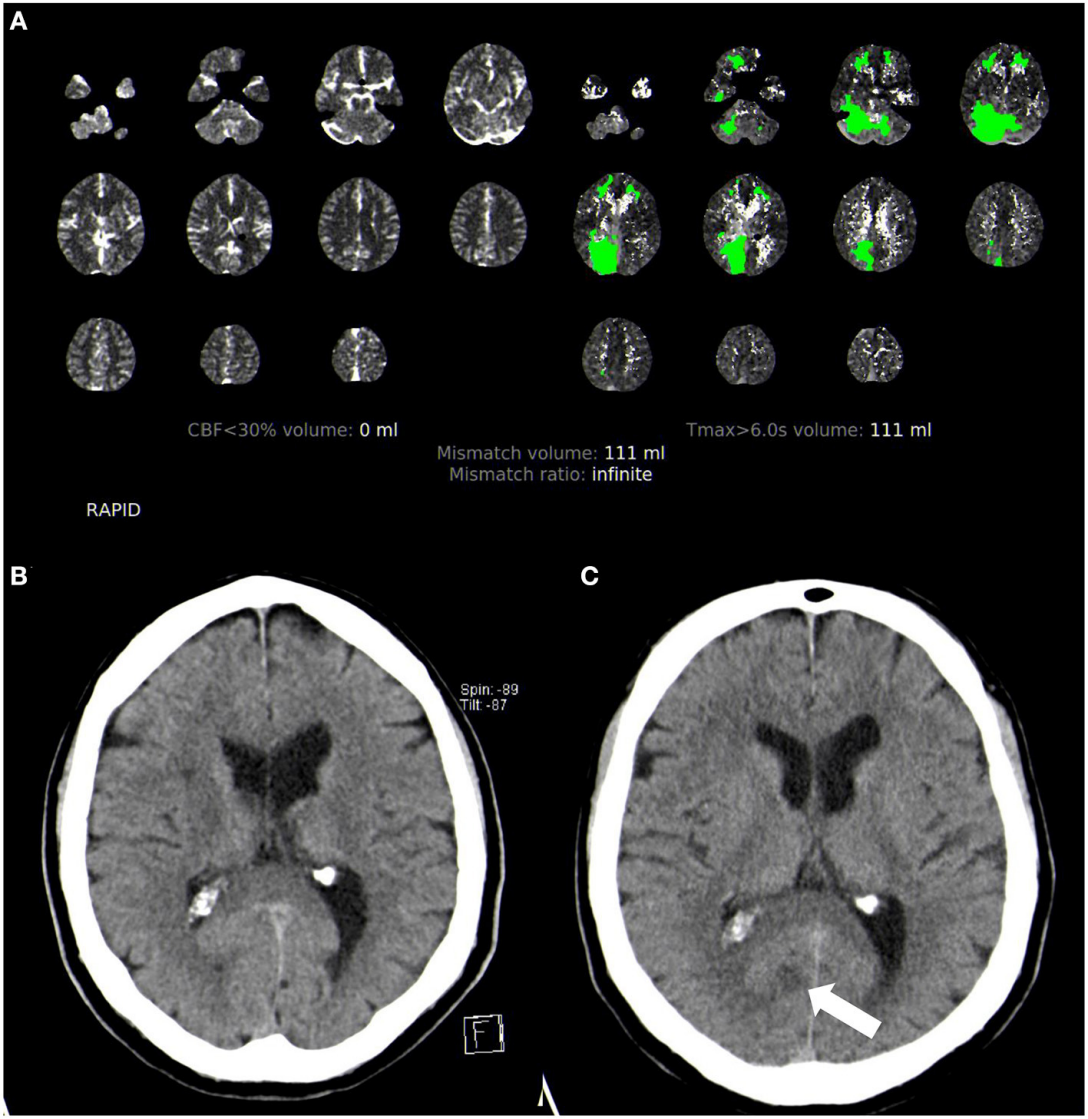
Acute multimodal CT and follow-up non-contrast CT imaging in posterior circulation stroke. (A) Perfusion CT analyzed with Rapid CTP. (B) Initial non-contrast CT showing no infarction. (C) Follow-up non-contrast CT with a visible small infarction in the posterior cerebral artery territory (arrow).
